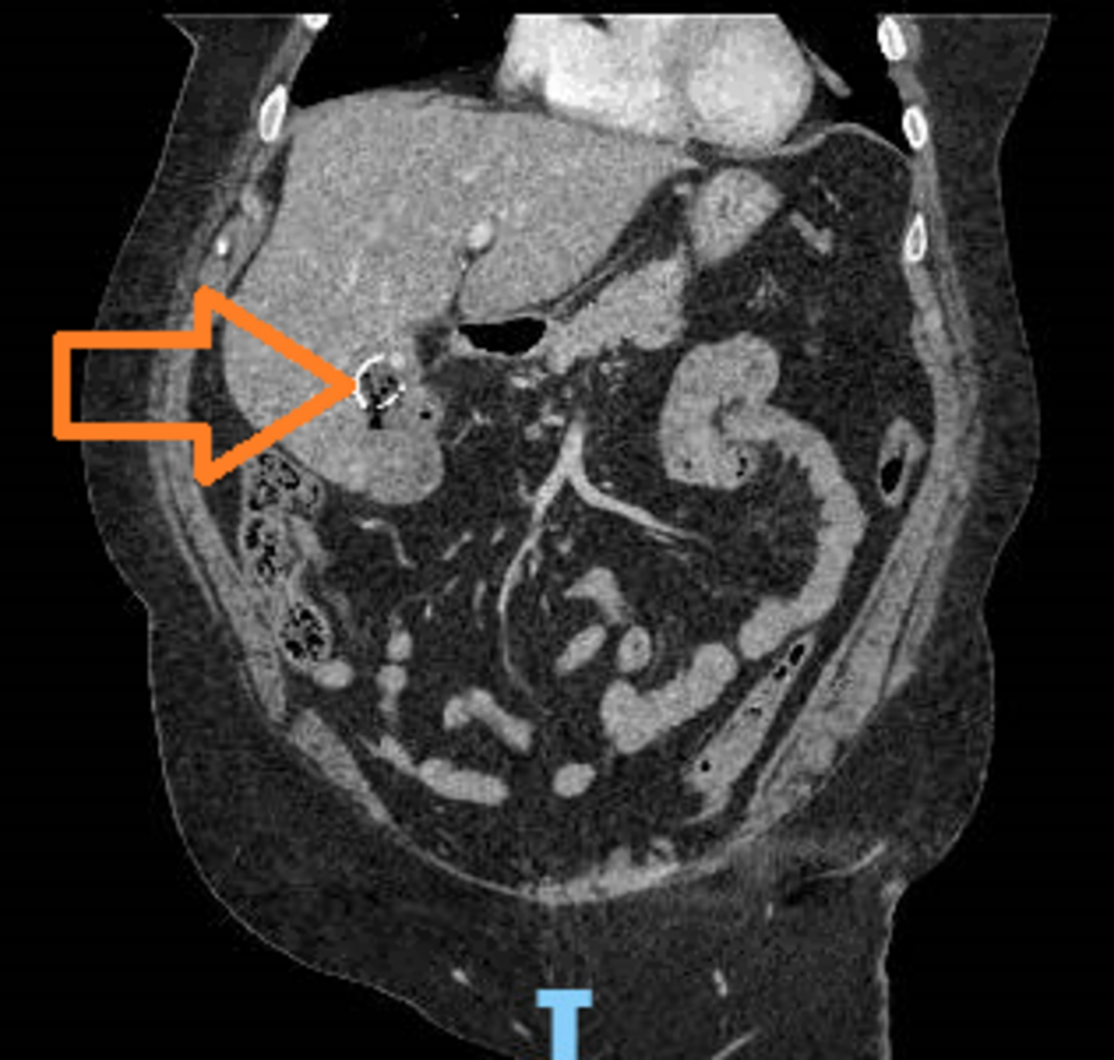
CT of the abdomen Arrowhead shows the porcelain gallbladder CT: computed tomography
